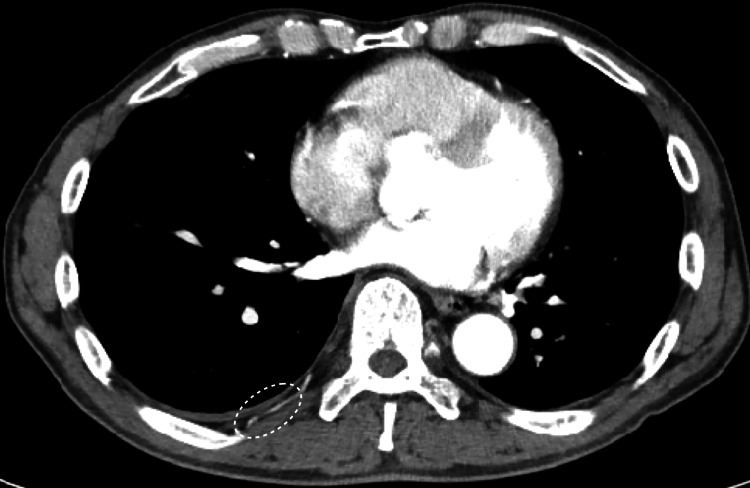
Follow-up CT imaging at nine months after discharge showing complete resolution of pleural effusion and intercostal lymphadenopathy (dotted circle).
